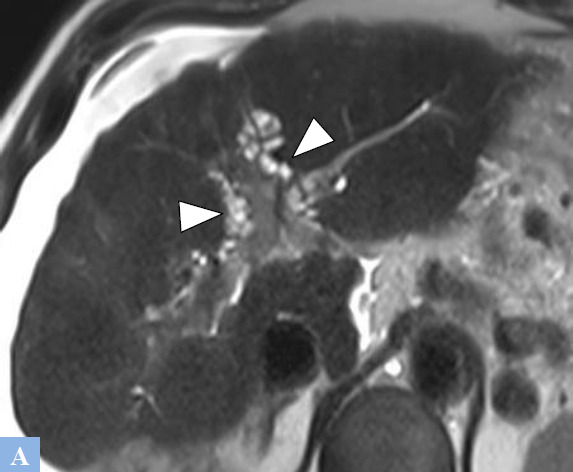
Peribiliary cysts in a patient with a history of alcohol-related cirrhosis. T2-weighted fat-suppressed MR image in the axial plane. Multiple centimeter-sized cystic lesions adjacent to the proximal bile ducts (arrowheads).
